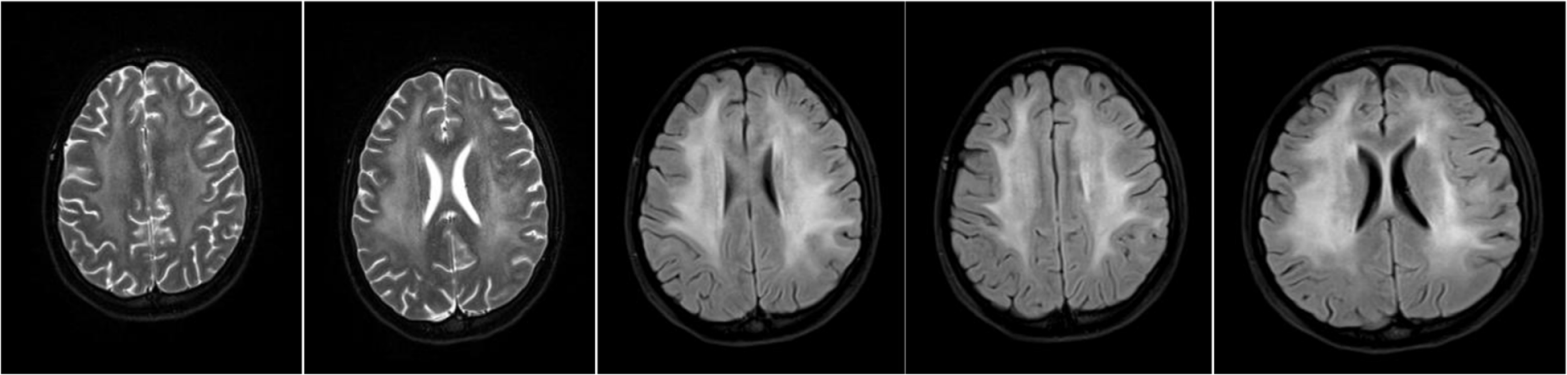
MRI brain with/without contrast
